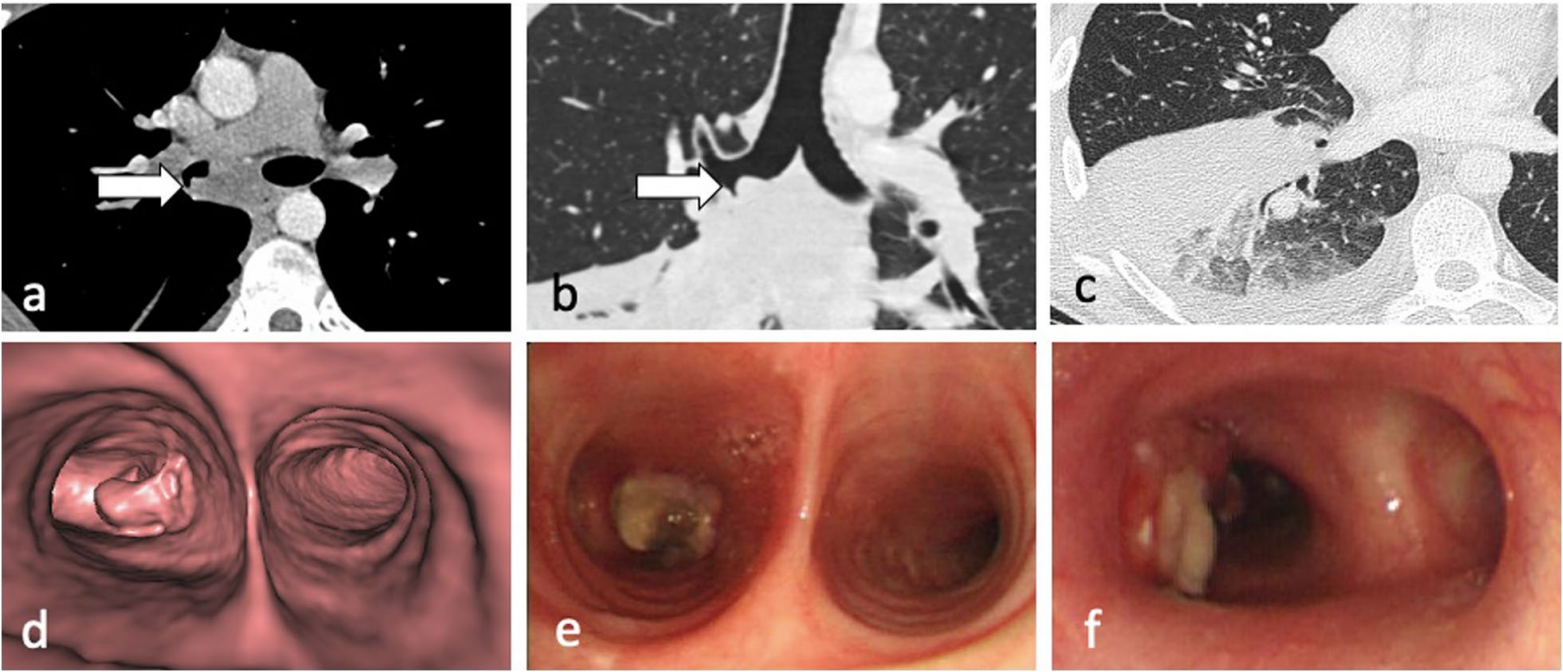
A 34-year-old male with Crohn’s disease presented with recurrent pyrexia and raised inflammatory markers. CT demonstrates a 29 mm subcarinal node with central low attenuation (mediastinal window, axial plane—a, arrow) invading into the right main bronchus (lung window, coronal plane—b, arrow). There is also right lower lobe consolidation and a small right pleural effusion (lung widow, axial plane—c). CT virtual bronchoscopy demonstrates the node protruding into the lumen of the right main bronchus (d) with corresponding bronchoscopy image (e). The patient underwent cryoprobe removal of the lymph node tissue successfully (bronchoscopy image—f)
